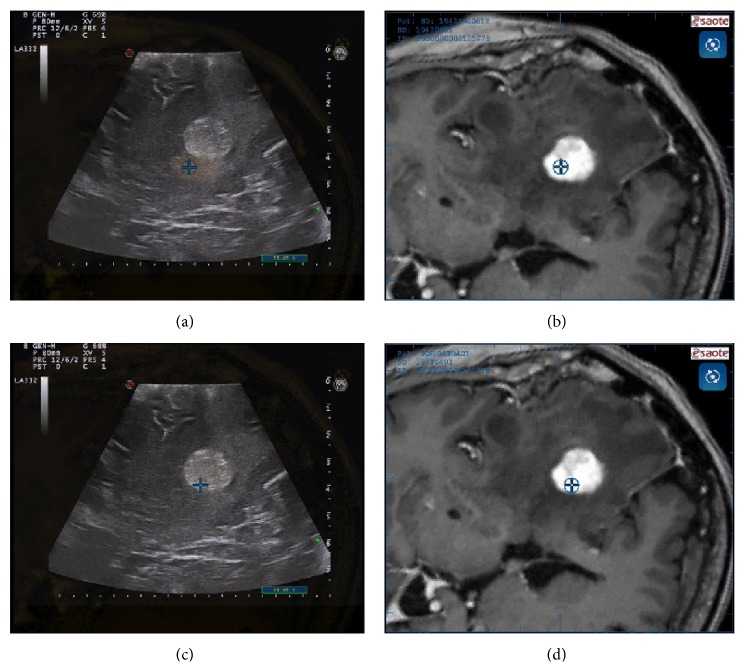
Brain shift correction. Every time a misalignment of ioUS and corresponding preoperative MRI (brain-shift) is appreciable, it is possible to realign the images in order to regain the system accuracy. In the upper panel, a misalignment in visible trough the fusion imaging. In the lower panel, the shift is fixed. In the right boxes, preoperative MRI are displayed.
